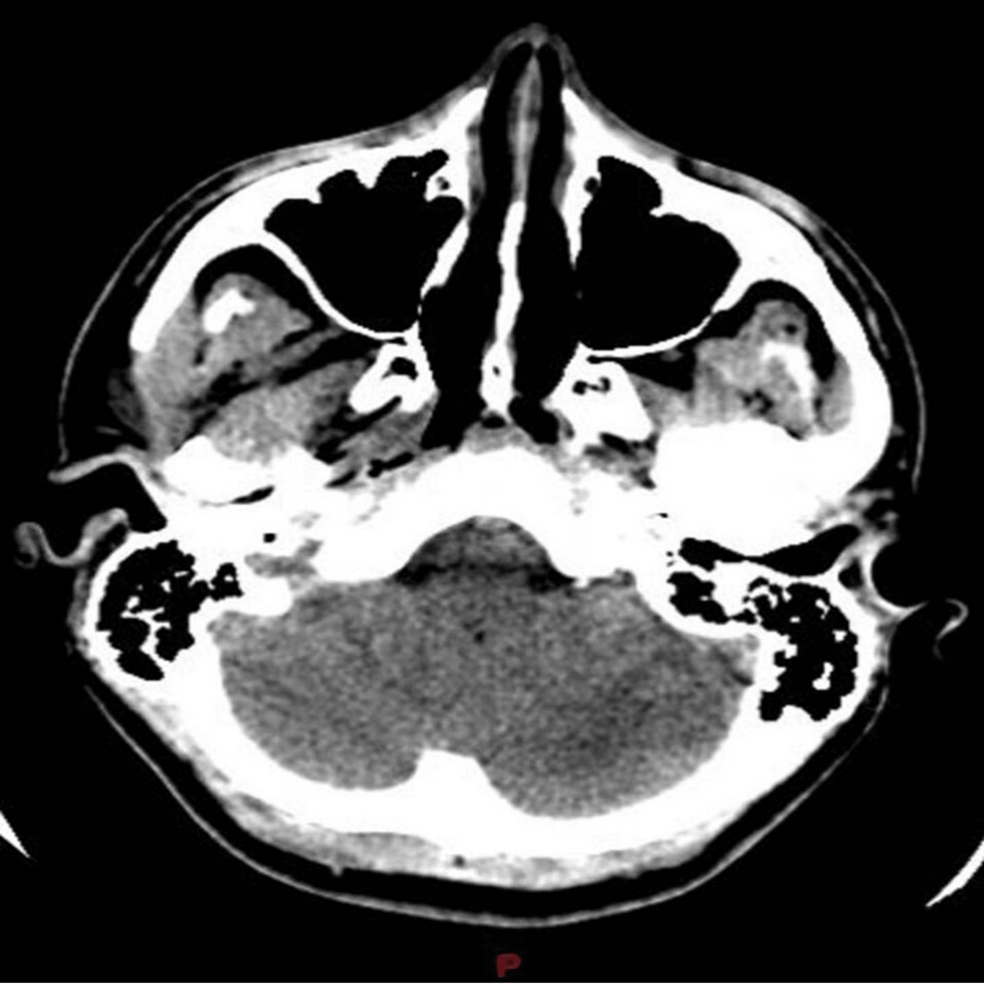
Brain CT scan at presentation
